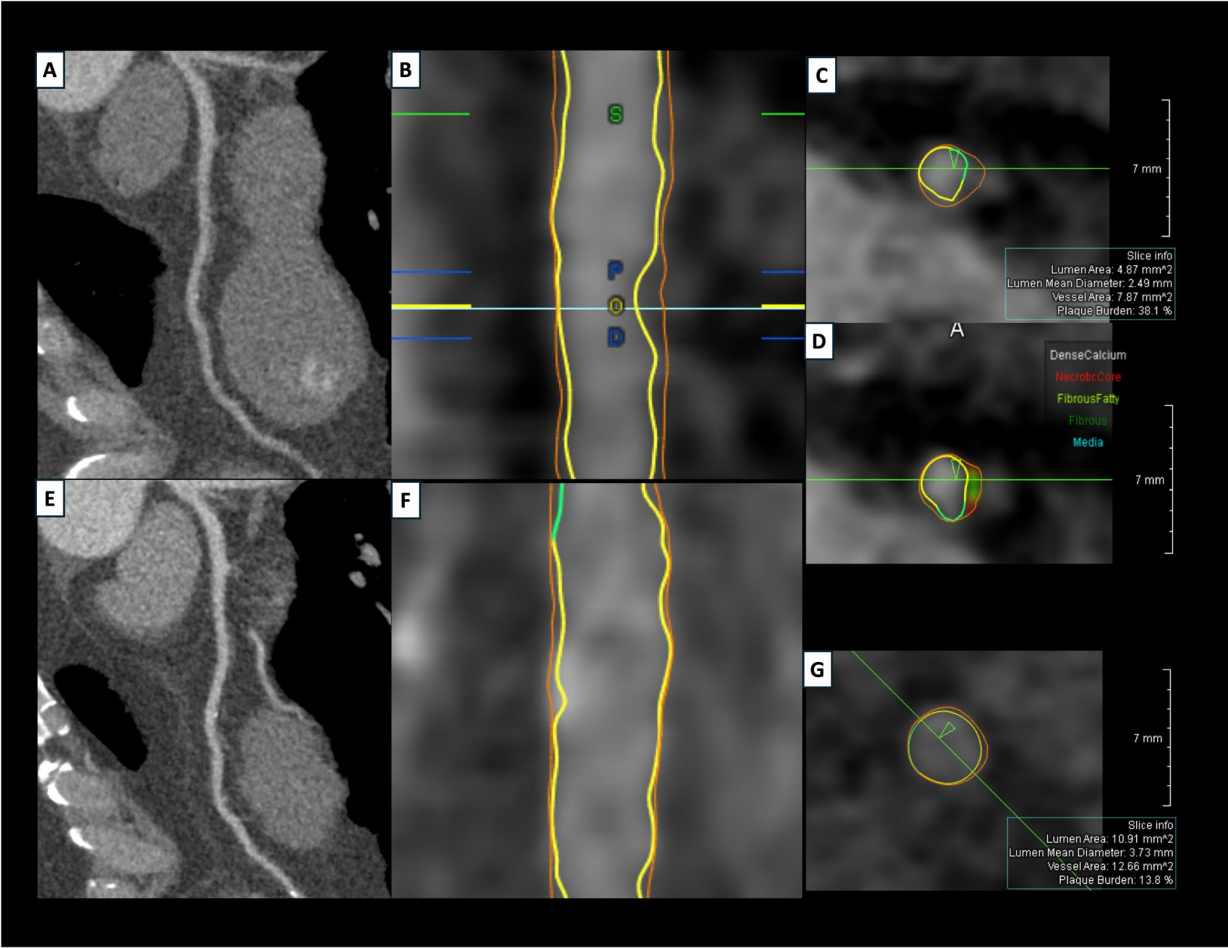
Case example of plaque regression in a patient treated with GLP-1Ra. A 46-year-old male patient presented with NSTE-ACS and underwent primary PCI on the right coronary artery. The initial CCTA (panels A–D) demonstrates a fibrous-fatty plaque in the mid-segment of the LAD, causing mild stenosis with a total plaque burden of 38.1%. At follow-up CCTA (panels E–G), advanced plaque analysis of the same LAD segment reveals minimal residual plaque (total plaque burden 13.8%) with near-complete restoration of the lumen area. *CCTA* coronary CT angiography, *LAD* left anterior descending artery
